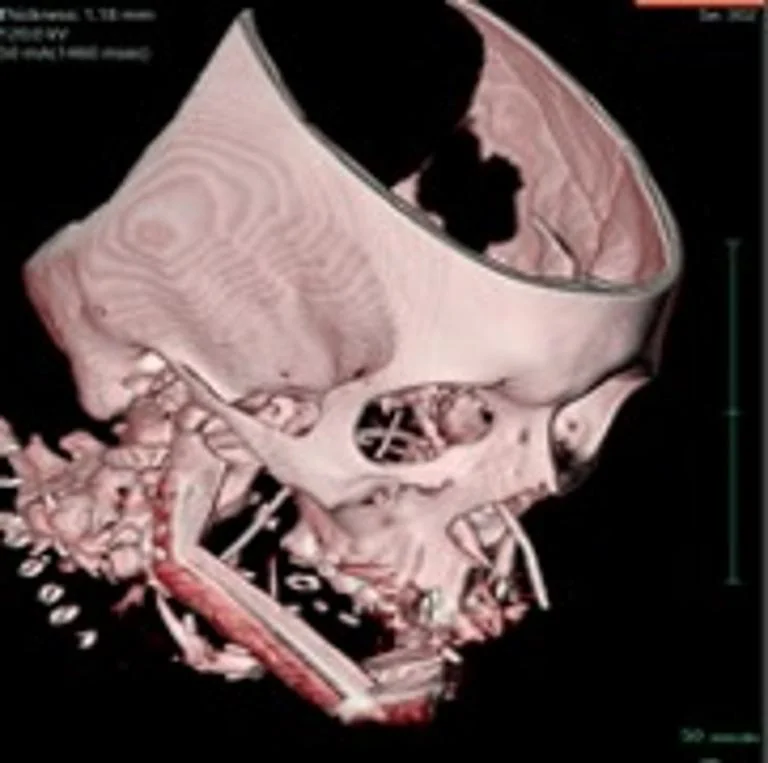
3D Post-operative CT Reconstruction showing the mandibular reconstruction
